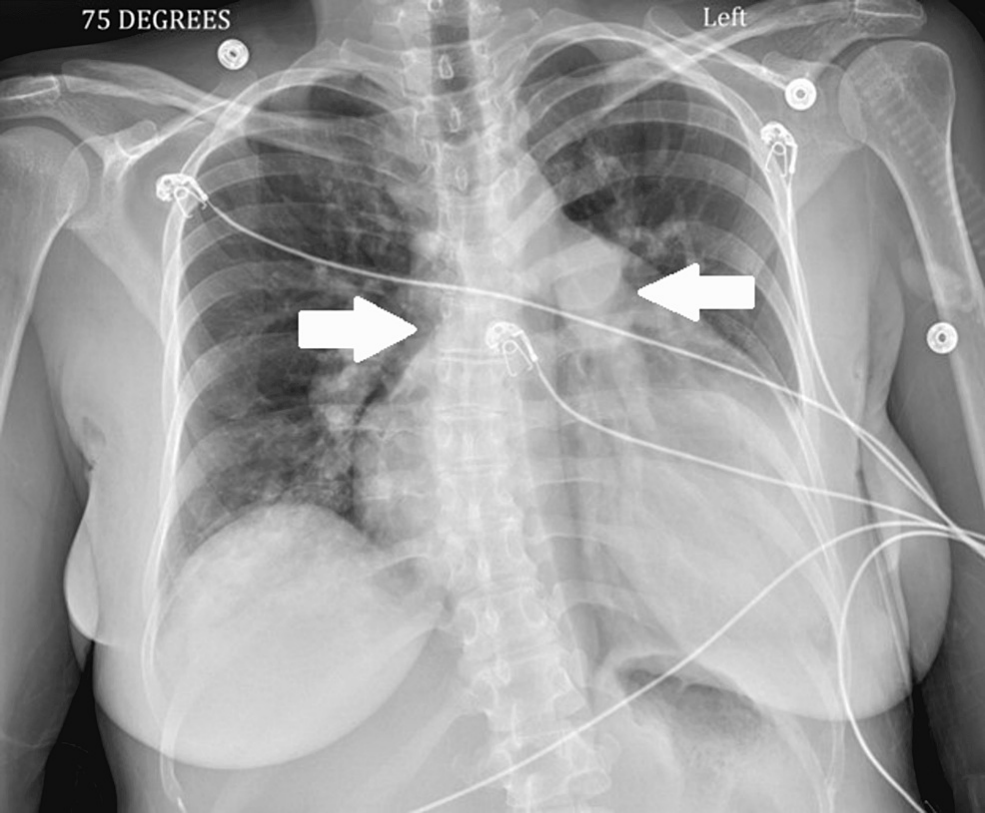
Case 1 chest X-ray demonstrating cardiomegaly and prominent pulmonary vasculature. Notice the ballooning of the right ventricle. Arrows indicate prominent pulmonary vasculature.
